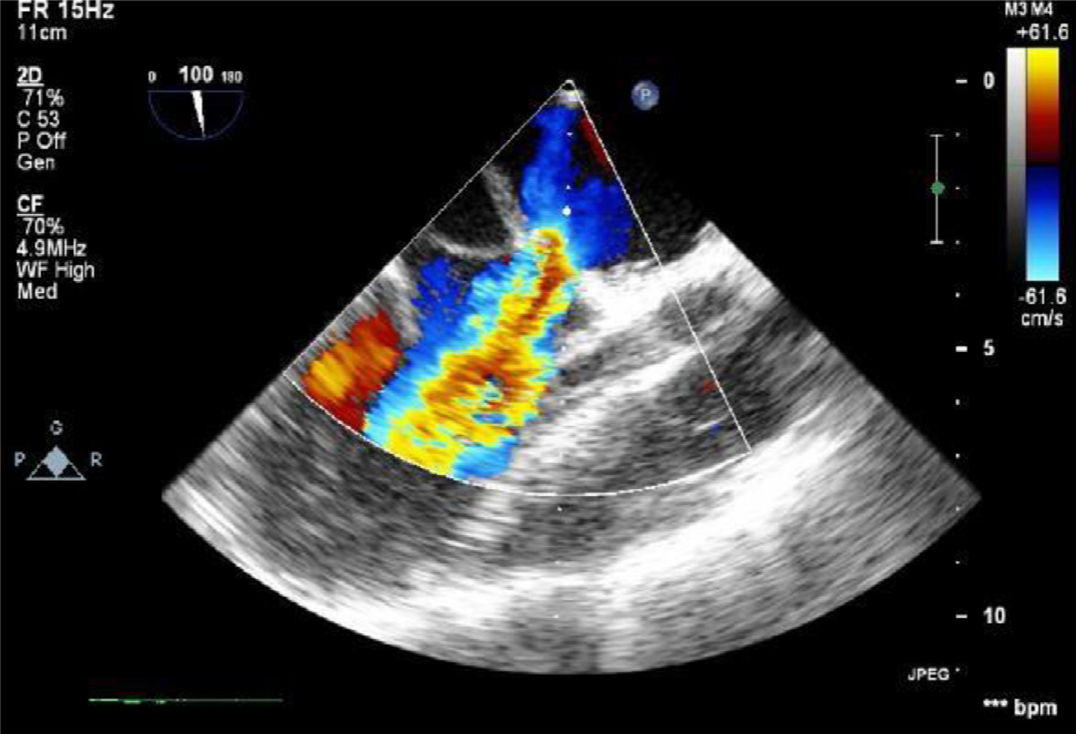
Transesophageal Echocardiography Image Long-axis view shows the turbulent flow in diastole across the opening in the fibromuscular membrane left between the proximal and distal left atrial cavities.
